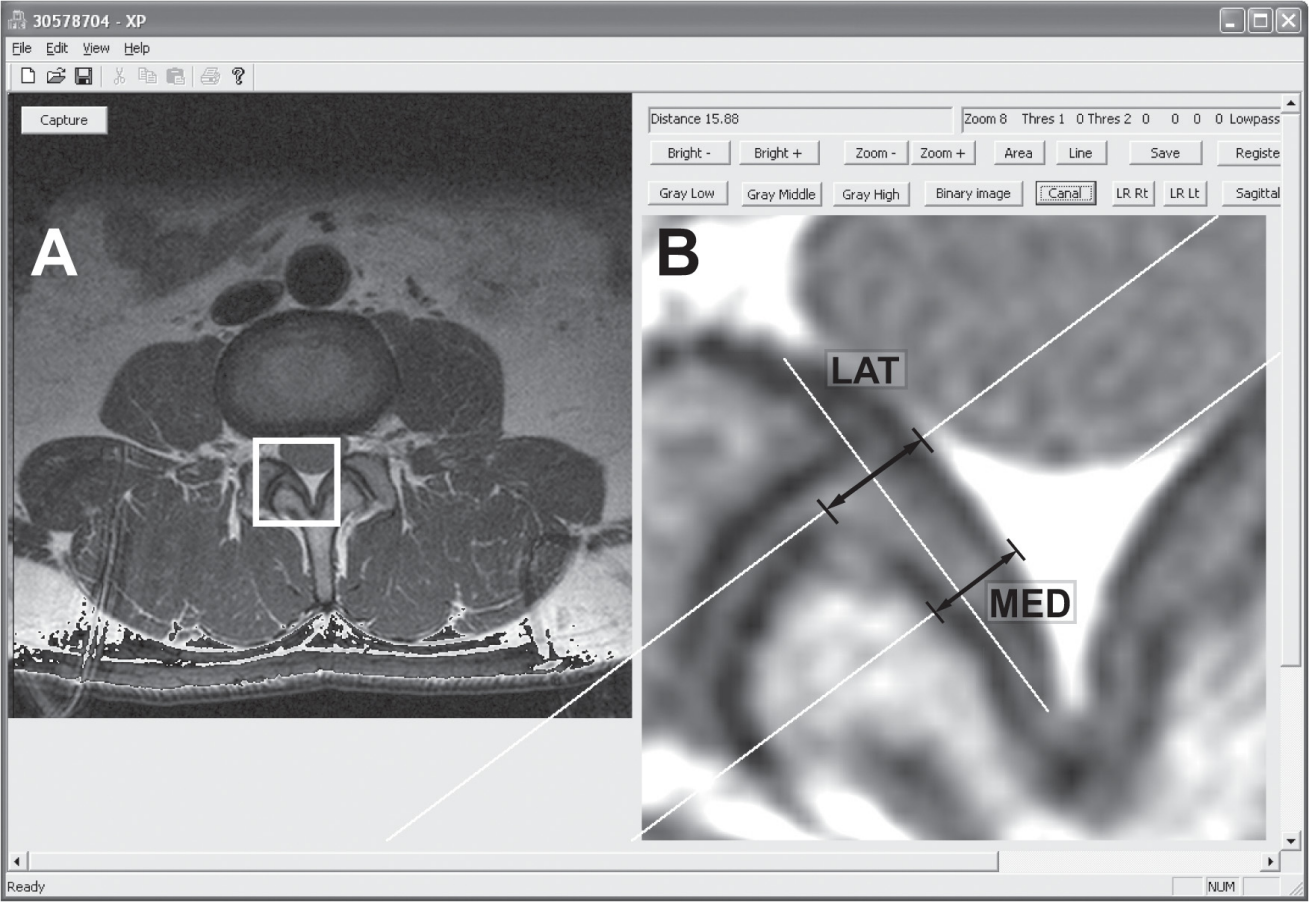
Screen capture of the image analysis program interface. (A) An original PD MR image. The region of interest box (shown as a white square) is selected for analysis. (B) Bilinear interpolation result showing an 800% enlarged image. Arrows indicate lateral thickness (LAT) and medial (MED) thickness.
